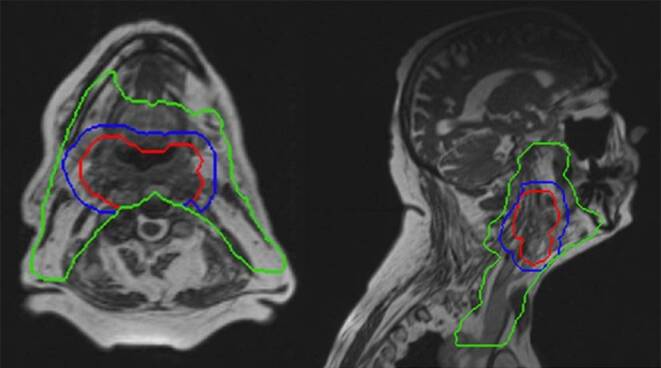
Planning MRI image and PTV contours of the clinical case used in the planning challenge. Three dose levels depicted in *red* (70 Gy), *blue* (59.4 Gy), and *green* (54 Gy). *MRI* magnetic resonance imaging, *PTV* planning target volume
